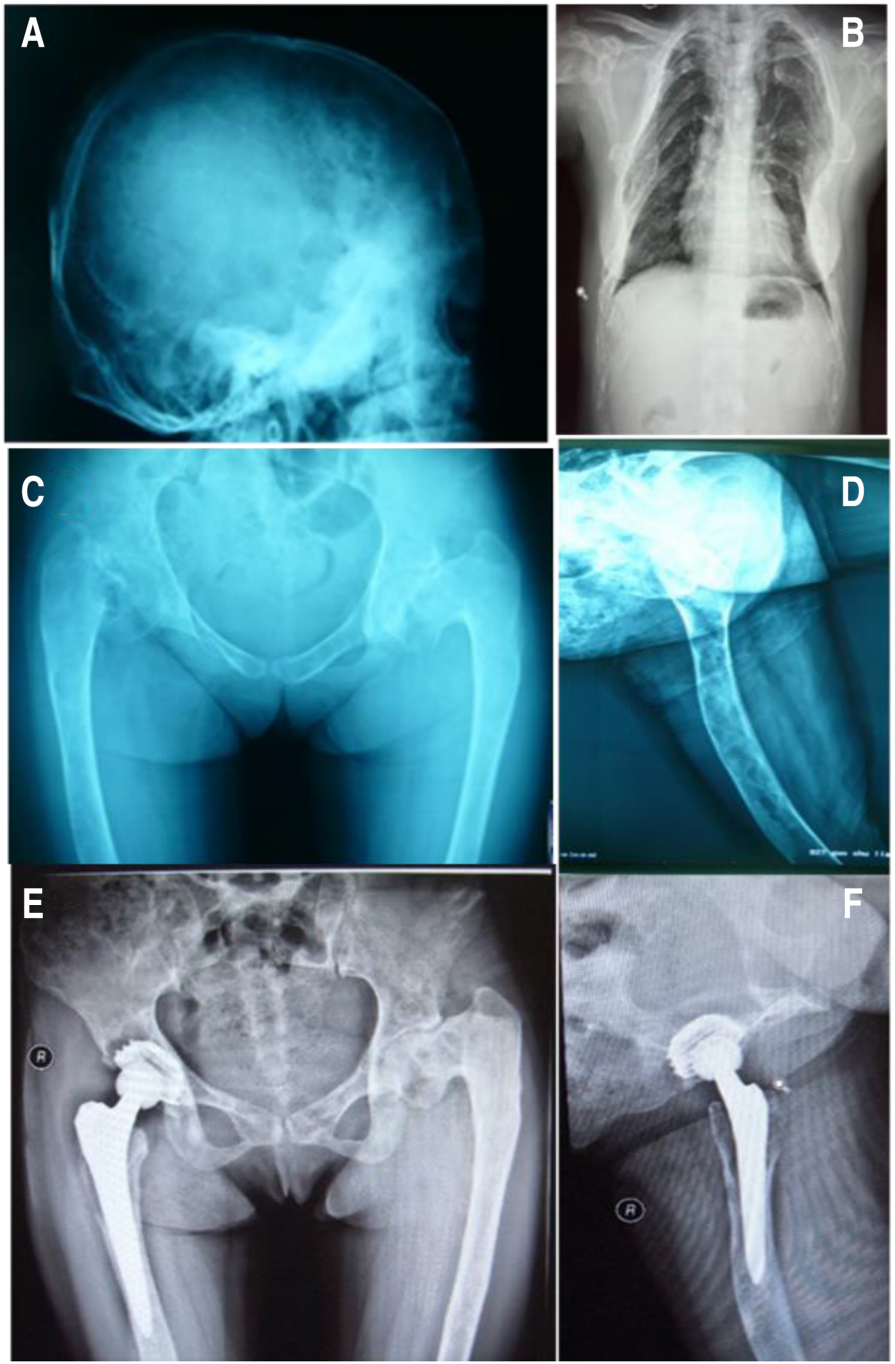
X-rays of the patient on initial admission. ABCD: Preoperative radiograph, EF: 22 months after right hip replacement.
